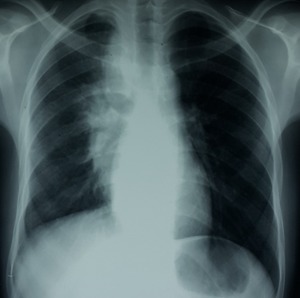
Radiographie thoracique de face montrant une opacité hétérogène suspecte médiastino-pulmonaire droite étendue
